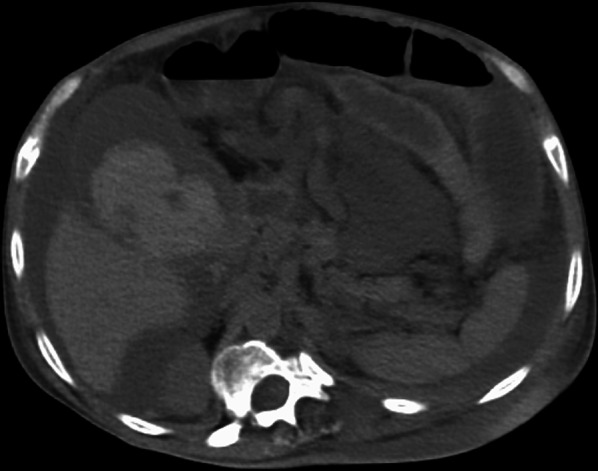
Computed tomography revealed high-density fluid collection within the peritoneal cavity and a distended gallbladder with high-density contents (blood)
